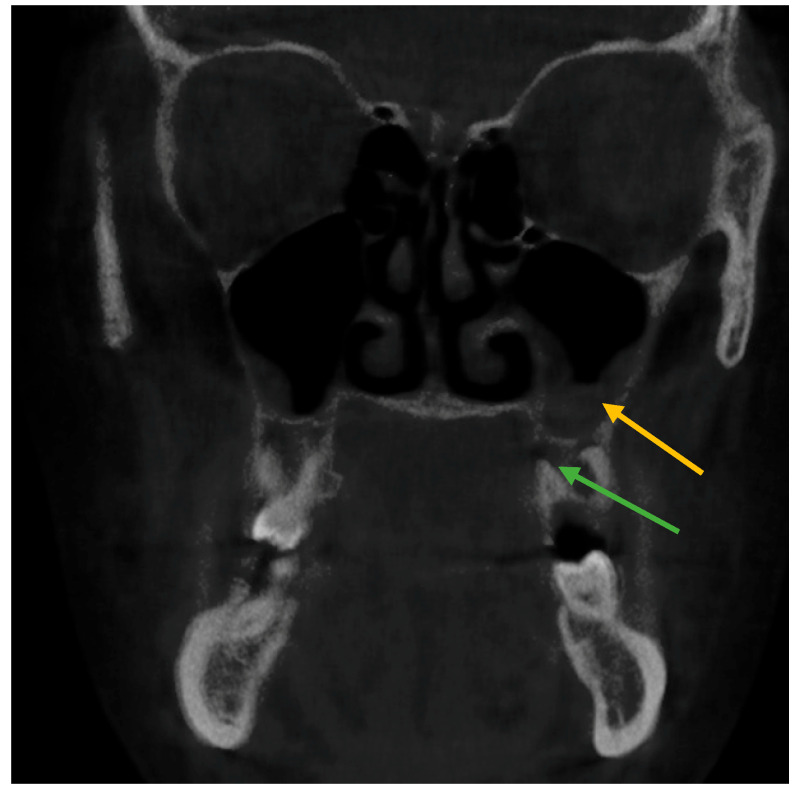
Changes in mucosal thickness in maxillary sinus observed in coronal view on CBCT imaging. (Yellow arrow shows mucosal thickening, green arrows show periapical infection).
